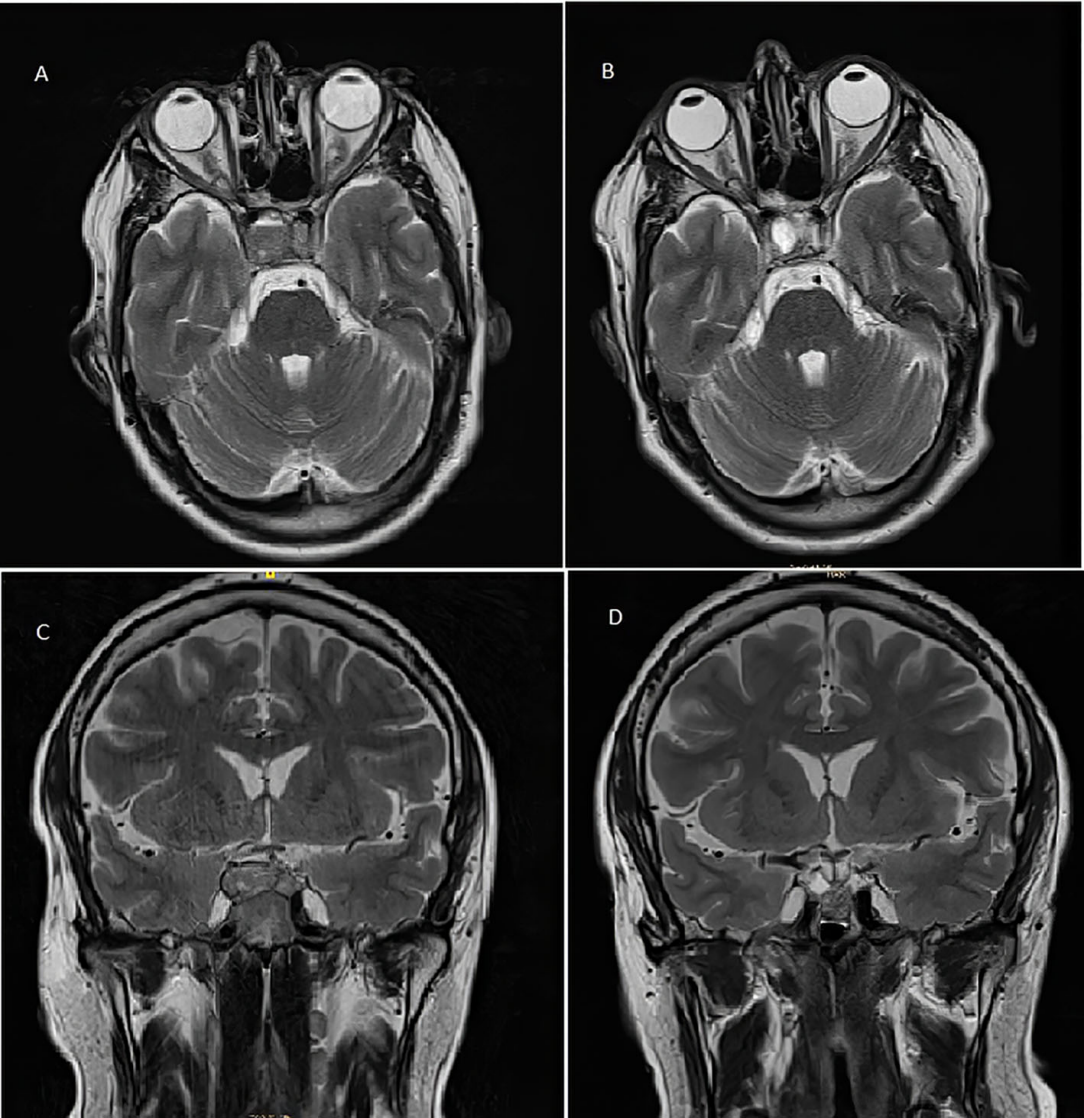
T2-weighted pituitary magnetic resonance imaging before (A, C) and after (B, D) treatment with capecitabine and temozolomide. (A) Pre-CAPTEM axial image. (B) Post-CAPTEM axial image shows a decrease in size of 65% with prominent cystic degeneration. (C) Pre-CAPTEM pituitary coronal image. (D) Post-CAPTEM coronal image shows a decrease in lesion.
